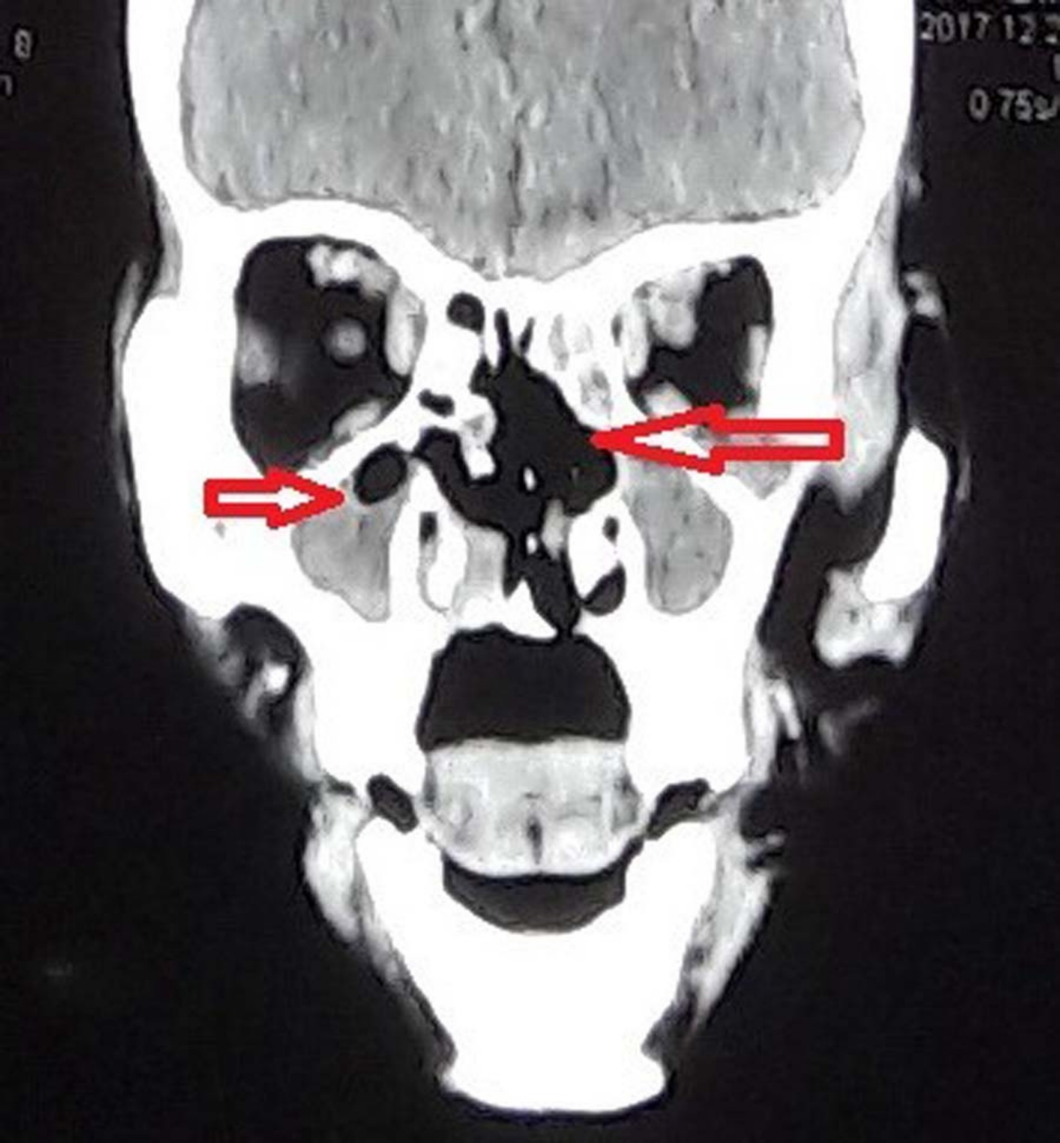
CT scan coronal view showing erosion of the nasal septum, turbinated and the hard palate along with bilateral maxillary sinusitis consistent with invasive fungal sinusitis.
